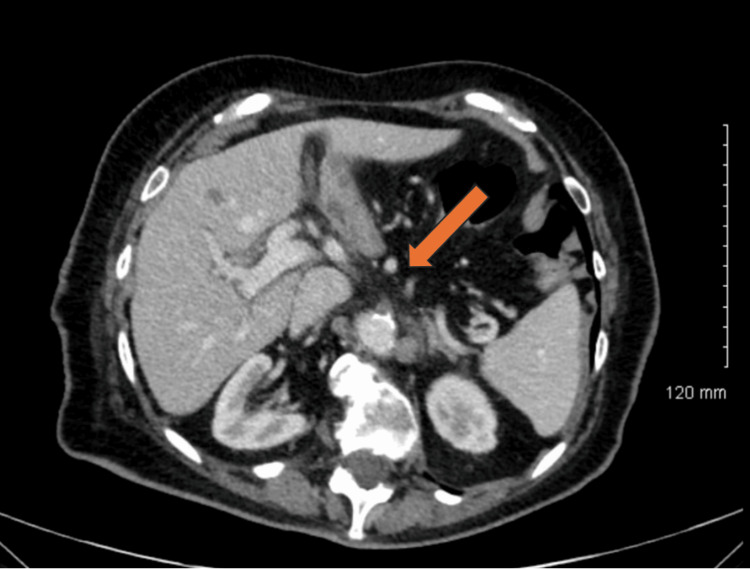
Axial section of a CT of the abdomen showing focal lack of enhancement in the proximal superior mesenteric artery.
